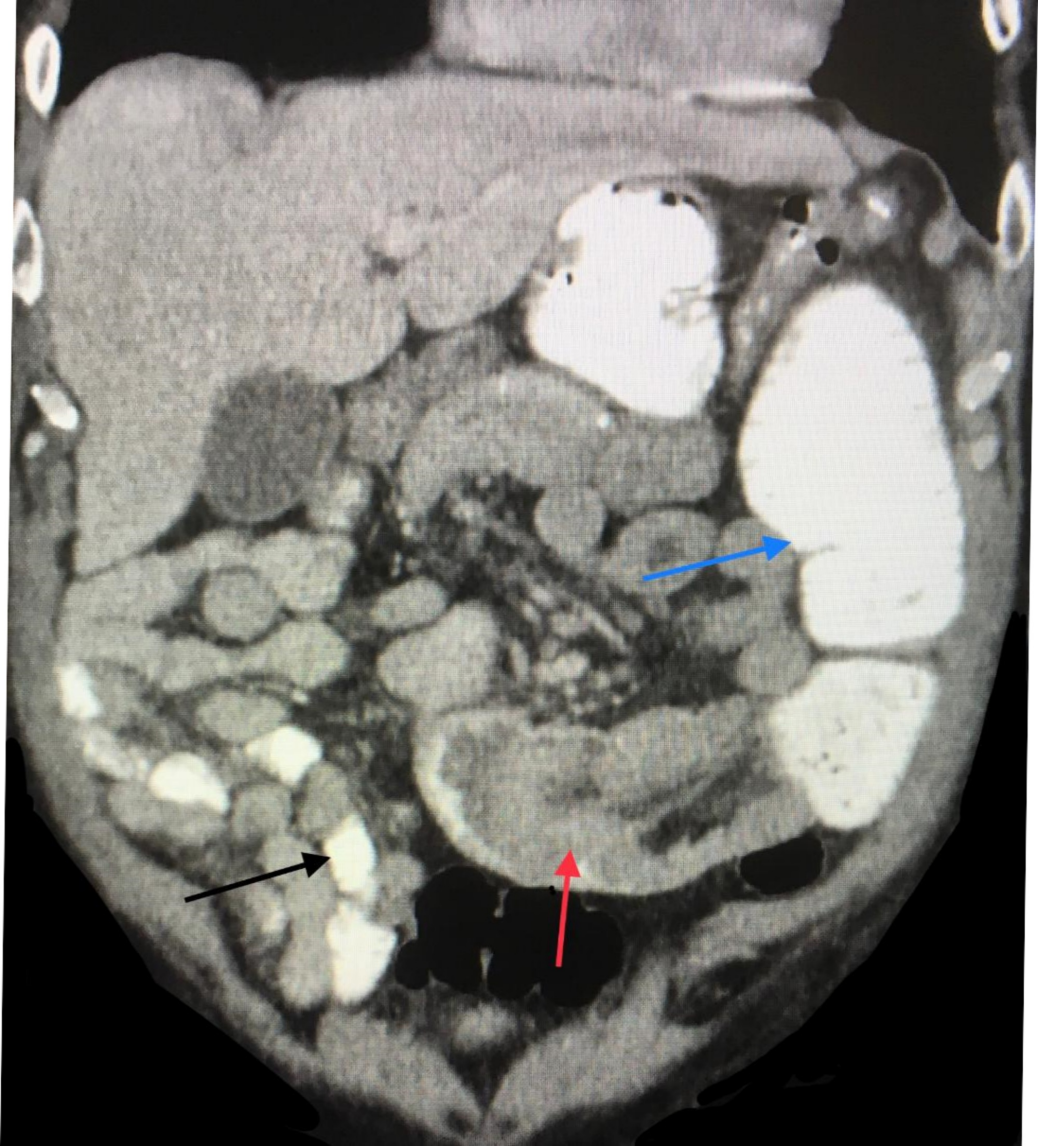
CT scan abdomen and pelvis Red arrow: jejuno-jejunal intussusception; Blue arrow: dilated bowel proximal to the obstruction; Black arrow: normal caliber bowel distal to the obstruction
